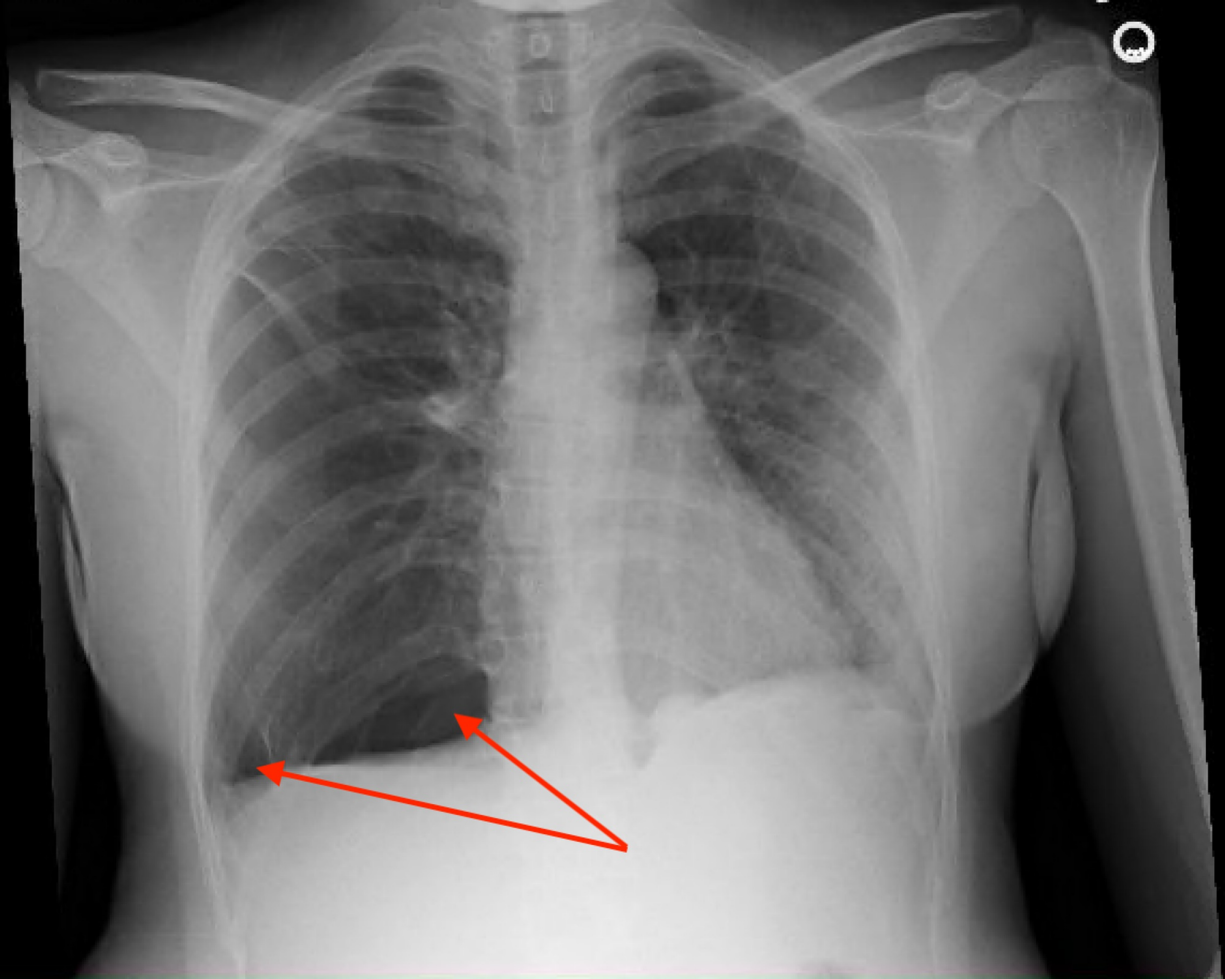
Chest X-ray showing right-sided basilar and lateral pneumothorax (red arrows).
